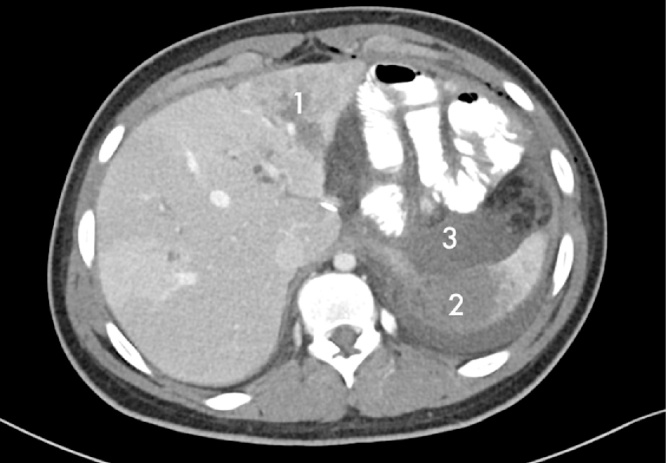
Patient 1. CT on second postop. day. Infarction in the left liver lobe (1) and the spleen (2) with neighbouring fluid collection (3).
